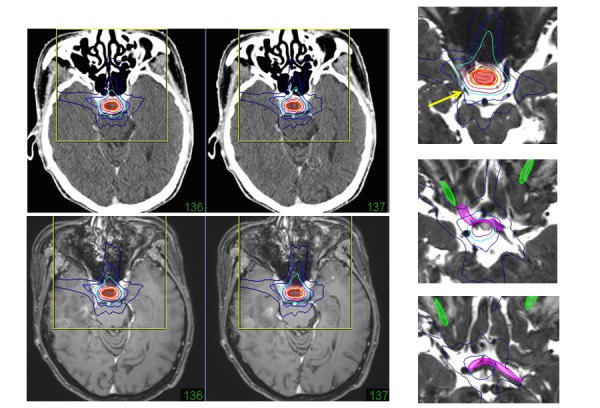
A sellar tumor and the surrounding structures. A Gd-enhanced MR image (lower left) fused to thin-sliced CT (upper left). Image fusion of the heavy T2-image showing the dose plan sparing the oculomotor nerve (yellow arrows), optic nerve (green), and optic chiasm (pink).
